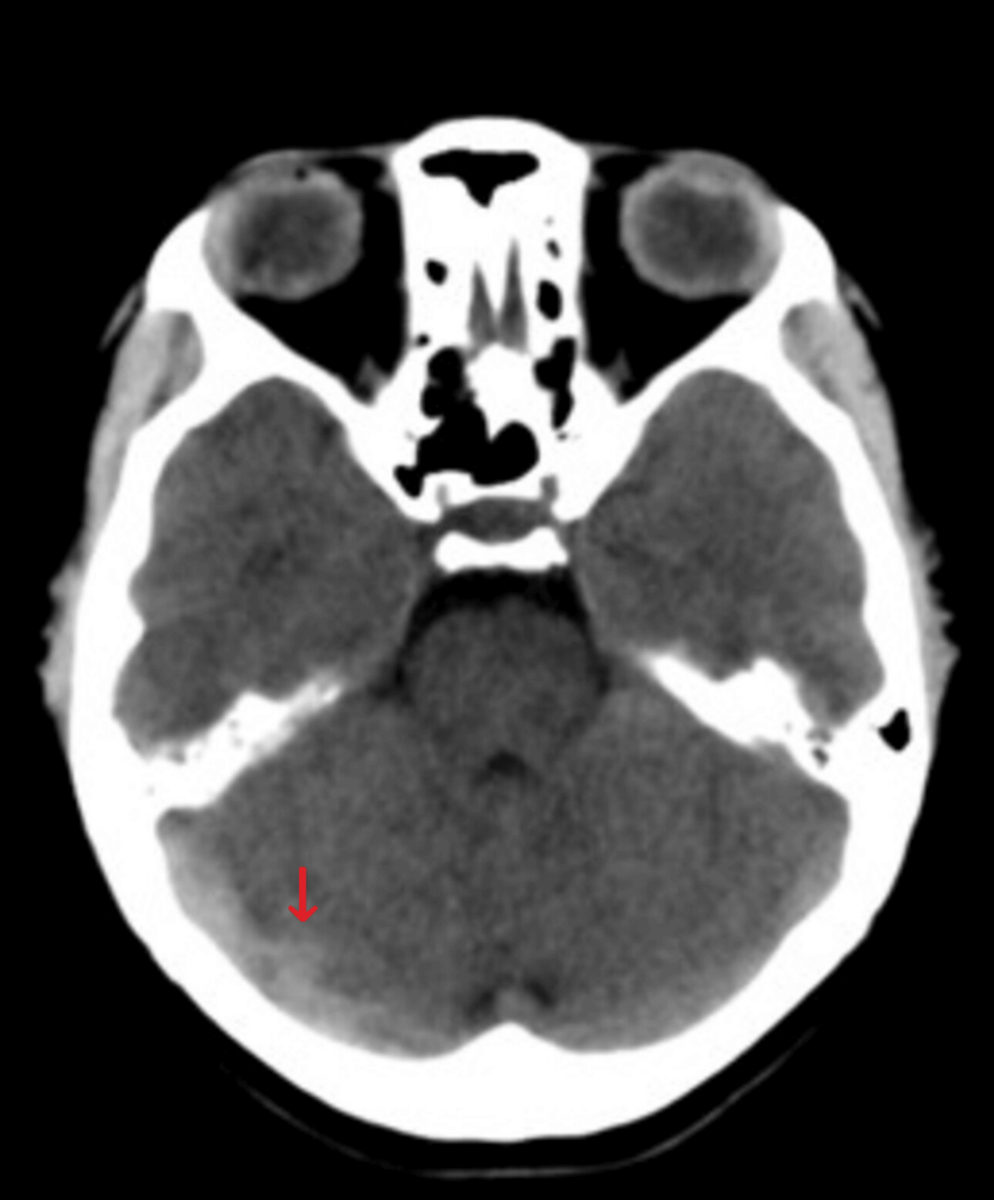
Axial CT scan of the single skull Hyperdensity in the right transverse sinus pathway
